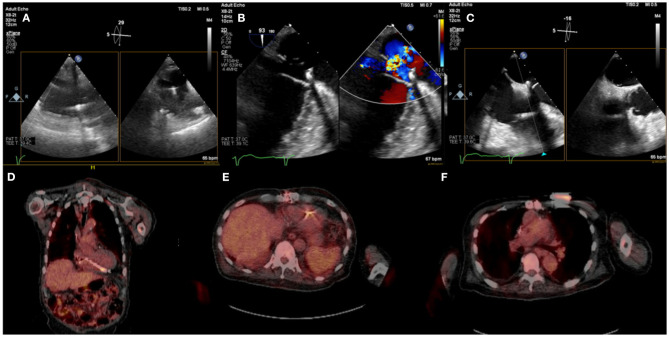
Eighty five year-old male with history ischemic cardiomyopathy s/p dual-chamber ICD for primary prevention, history of CABG and mitral valve repair presented with persistent MRSA bacteremia. TEE showed small linear echodensities attached to right atrial lead (A), multiple small echodensities attached to mitral ring and moderate mitral regurgitation (B). The RV showed no vegetations with normal tricuspid valve (C). ^18^F-FDG PET-CT was obtained to assess extent of infection and showed focal uptake along the RV lead (D,E). Left chest wall ICD showed focal uptake but no pocket uptake to suggest pocket infection (F). The patient was deemed to have a prohibitive risk for redo cardiac surgery and was treated medically. He passed away 2 months after the diagnosis of CIED systemic infection with MRSA endocarditis.
